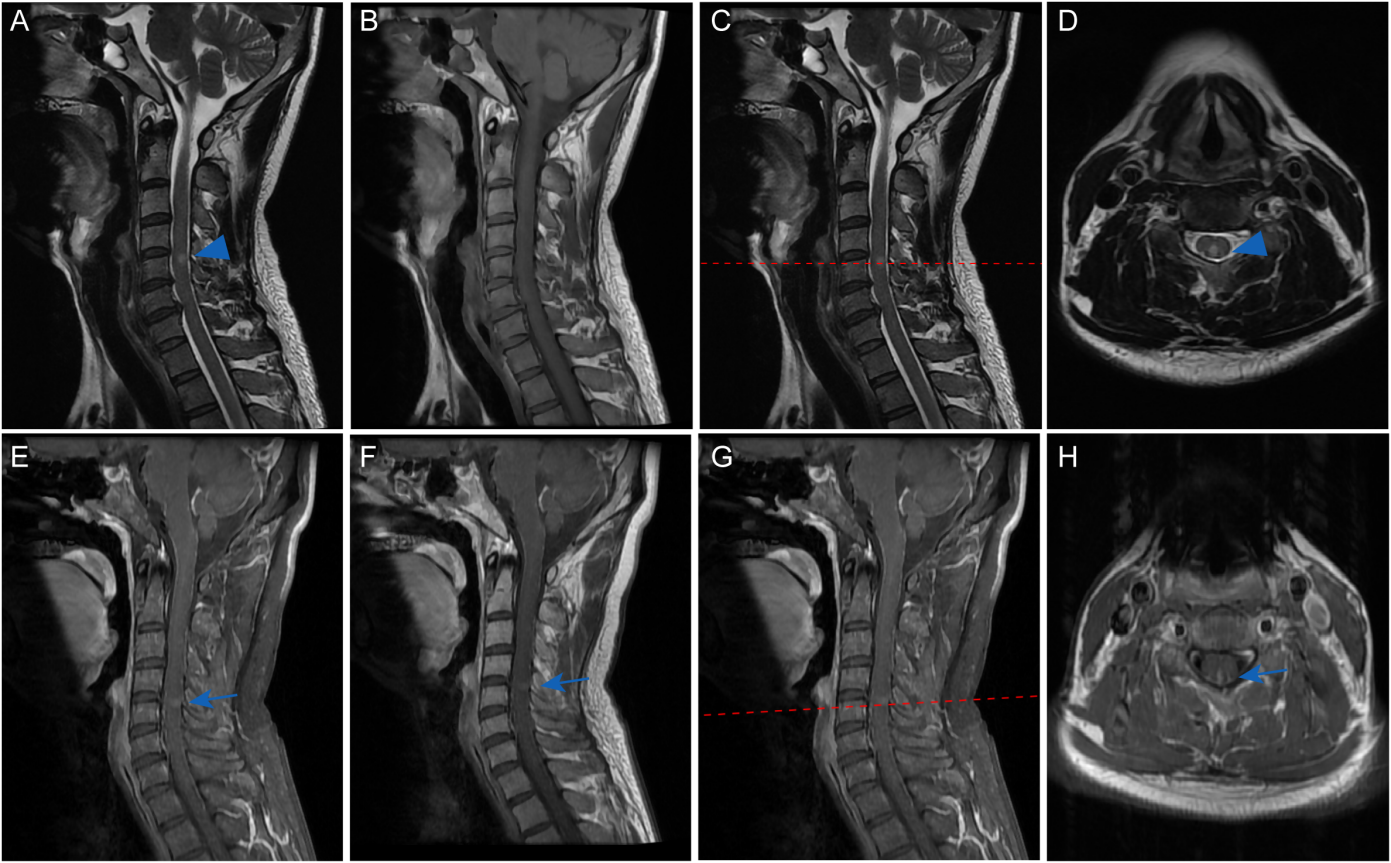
Cervical spinal MRI of the patient. Sagittal T2-weighted imaging demonstrated a discrete intramedullary hyperintensity spanning the C4-C5 levels (blue arrowhead, (A), which mainly located in the posterior part on axial views (blue arrowhead, (C, D). Corresponding T1-weighted sequences revealed iso-intensity without mass effect or cord expansion (B). Contrast-enhanced sequences exhibited subtle linear enhancement along the posterior lesion margin on both sagittal (blue arrow, (E-G) and axial views (blue arrow, H). Mild leptomeningeal enhancement was noted at the cervicomedullary junction (E-G).
